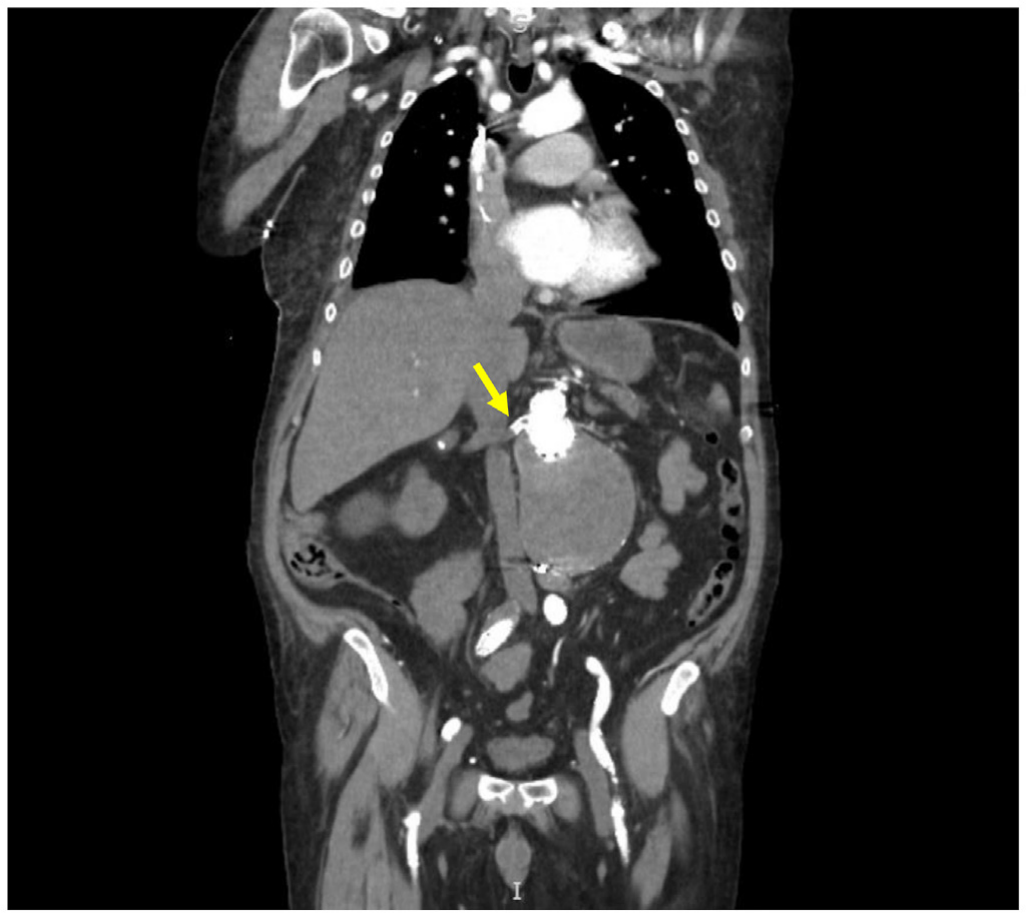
A computed tomography scan with intravenous contrast medium of the abdomen showing an occluded proximal right renal artery (*yellow arrow*).
